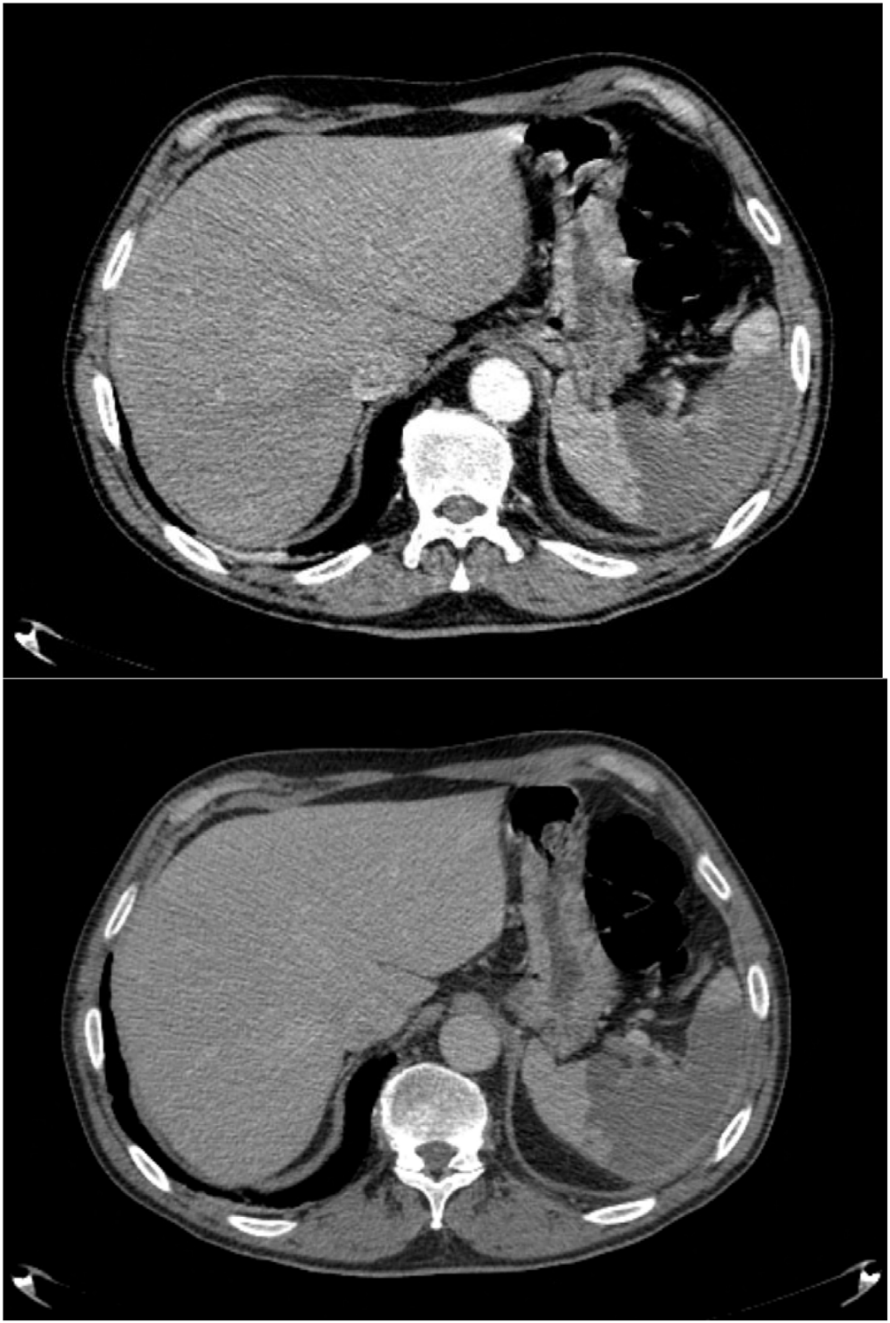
Axial CTA images showing hypodense areas throughout the spleen consistent with splenic infarctions.
